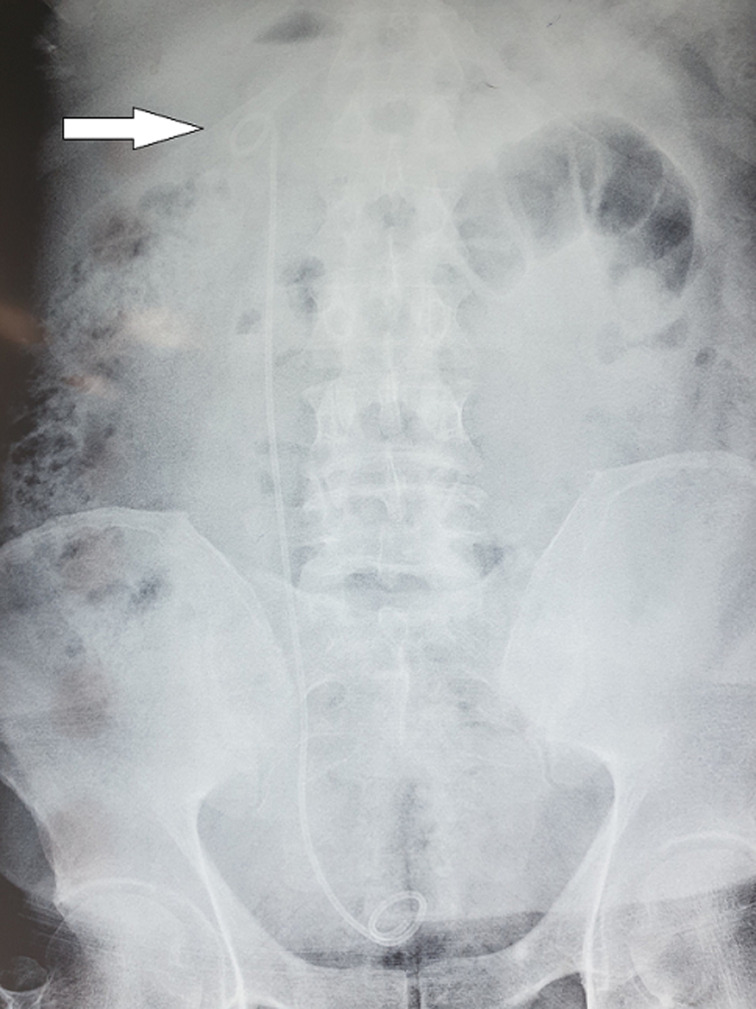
plain radiography X-ray before stent removal: no knotting in the stent (arrow)
